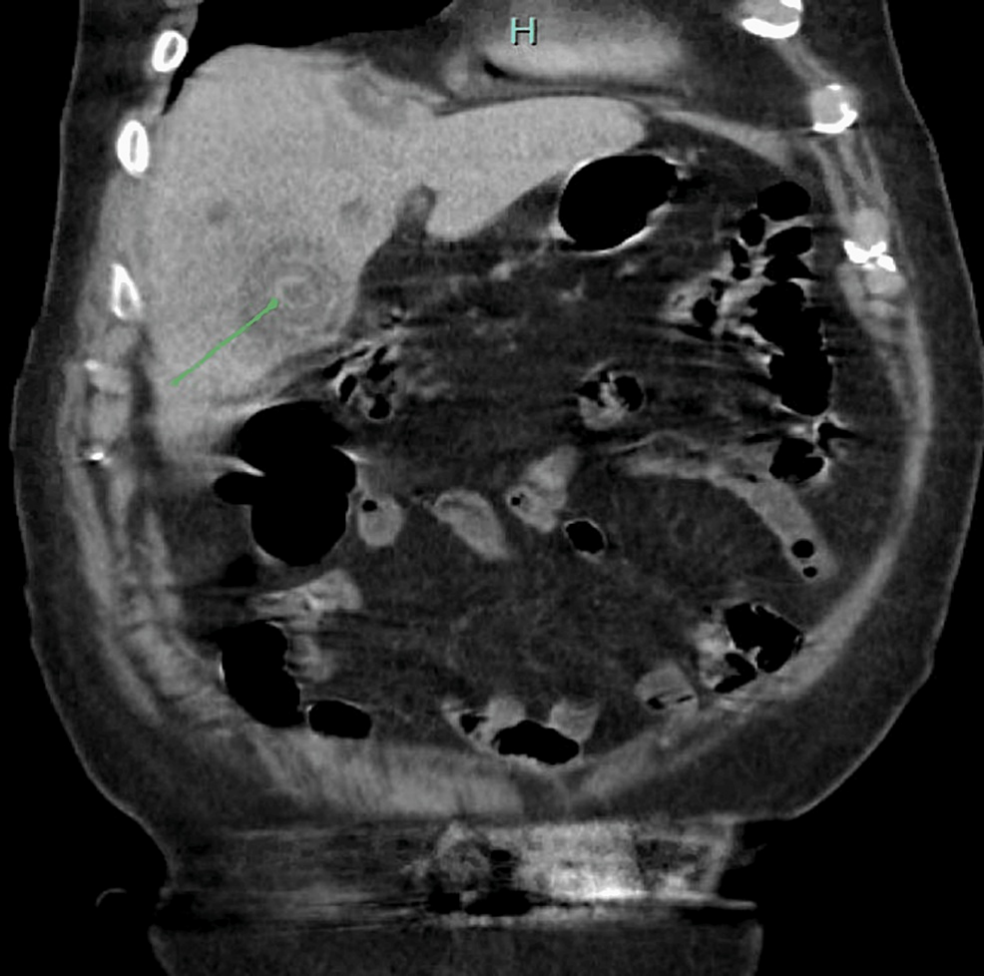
CT of the abdomen and pelvis with contrast in coronal view shows questionable mass versus sludge noted within the gallbladder lumen (green arrow).
